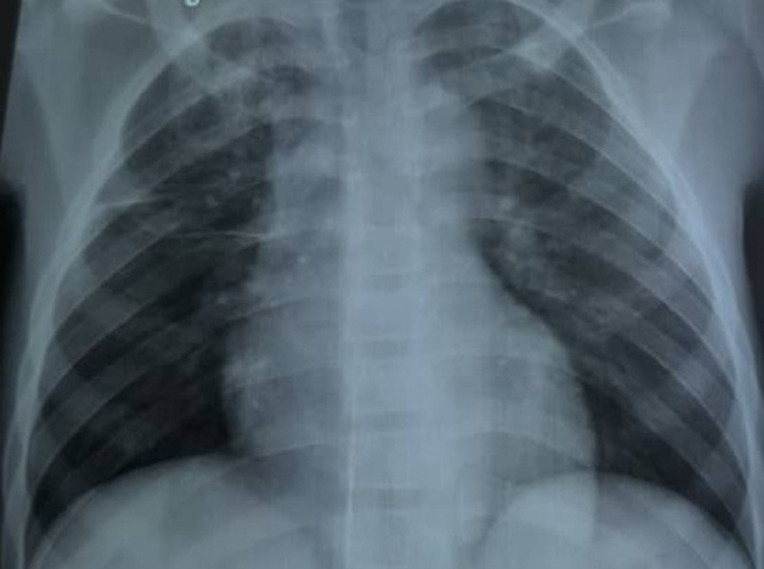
radiographique montrant des adénopathies hilaires et une excavation apicale à droite
